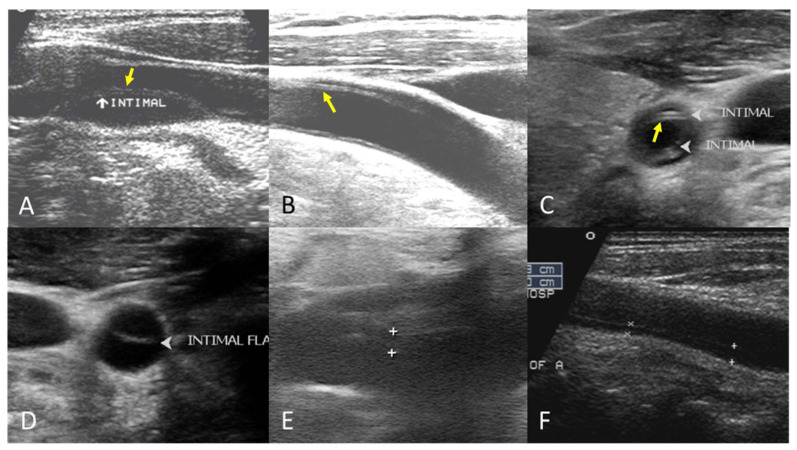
Double lumen with intimal flap with train-line pattern (A–C) and without train-line pattern (D–F). The ultrasound image was obtained using a 4× magnification setting. Yellow arrows indicate the “train line”. The train-line pattern is characterized by two closely positioned, hyperechoic parallel linear reflections overlying a thickened vessel wall. Symbols such as “×”, “+”, arrows, and arrowheads were generated by the ultrasound machine and used by sonographers to mark regions of interest (ROIs) where the intimal flap was located.
